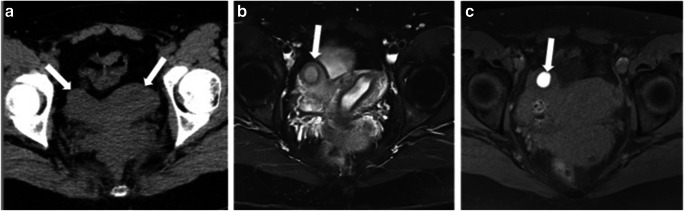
Role of CT in FGTCA assessment. a Non-reported FGTCA in CT examination performed for renal colic. Unenhanced CT shows an abnormal uterine contour (arrows), suggesting a uterine malformation. The patient returned some years later for pelvic MRI in the context of infertility work-up. T2W FS (b) shows a right rudimentary horn (arrow) not communicating with the main uterine cavity. T1W FS (c) depicts high SI in the rudimentary cavity, in keeping with haematometra (arrow). It is important to keep a high grade of suspicion, as early diagnosis of FGTCA may help to avoid prolonged symptomatic periods and the complications that may subsequently arise, such as infertility and endometriosis
